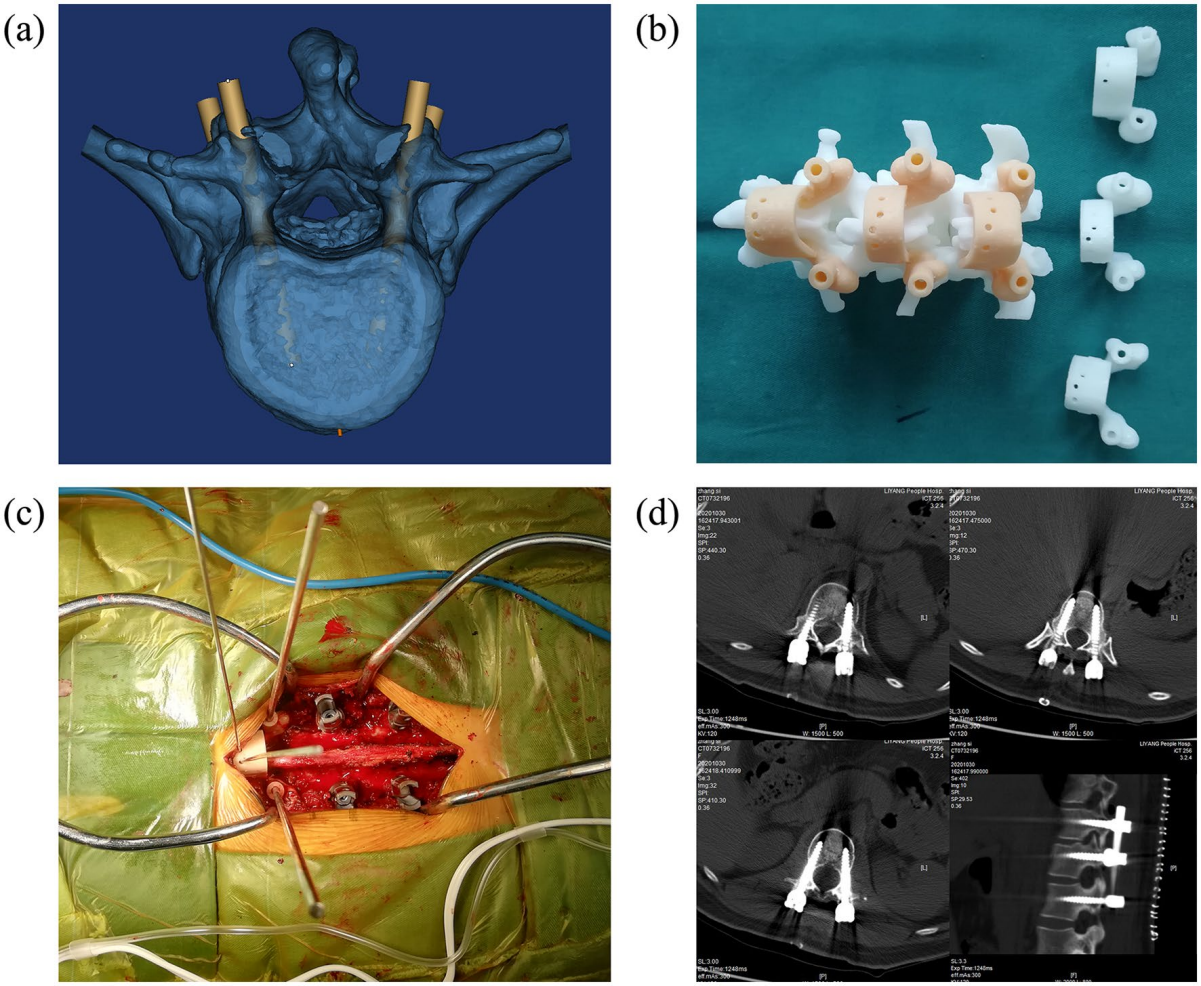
Clinical application of PSNT: (a) an established pedicle screw channel in Mimics software; (b) PSNTs were designed in 3-Matic software. Then, the PSNTs were printed with PLA and resin respectively and were sterilized by the plasma; (c) during the operation, the PSNTs matched the bone surface well, and the screw channel was positioned accurately; (d) Postoperative CT showed that the position of 6 pedicle screws was excellent.
